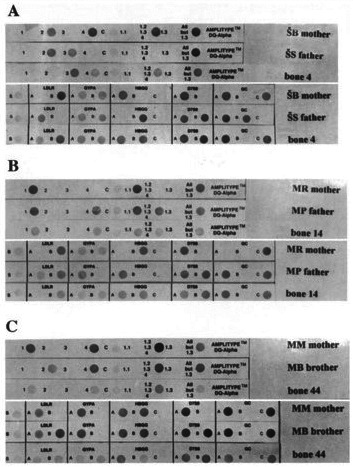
Results of the first (historical) DNA identification of skeletal remains found in Kupres mass graves by AmpliType^®^ PM+DQA1 PCR Amplification and Typing Kit (PerkinElmer Corp., Norwalk, CT, USA).
